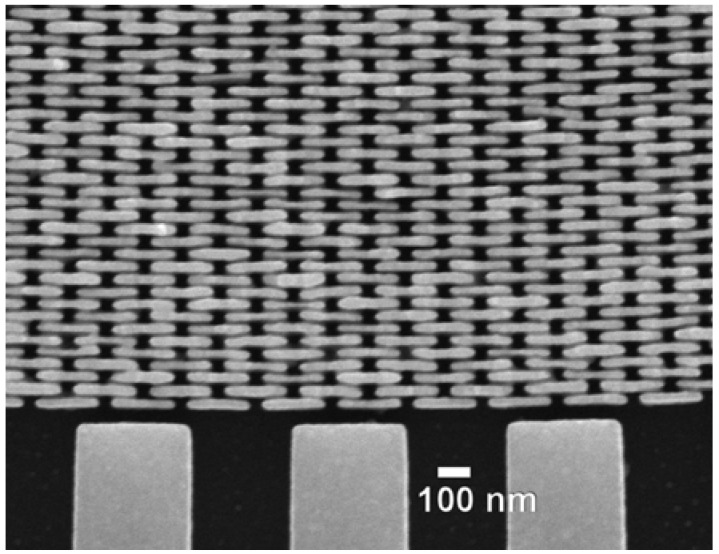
The region near the edge of the hard-X-ray FZPs of Figure 20. The “broken ring” pattern is designed to enhance the mechanical stability for high aspect ratios.
